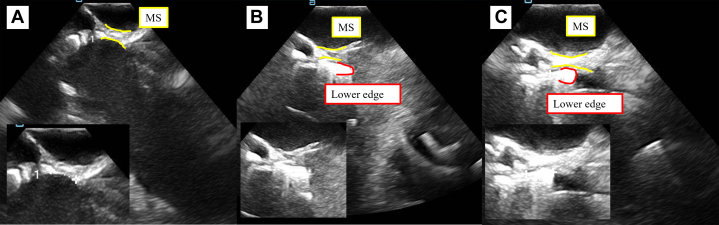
Transjugular Intracardiac Echocardiograms of Valve Deployment (A) Predeployment intracardiac echocardiogram showing the membranous septum (MS) length measured at 4.7 mm, which was consistent with the preprocedural computed tomography measurement. (B) First valve deployment attempt demonstrating the valve's lower edge extending beyond the MS and reaching the muscular septum. (C) Final deployment position showing the valve's lower edge fixed at a depth of 4.0 mm from the annulus, without extending beyond the MS.
